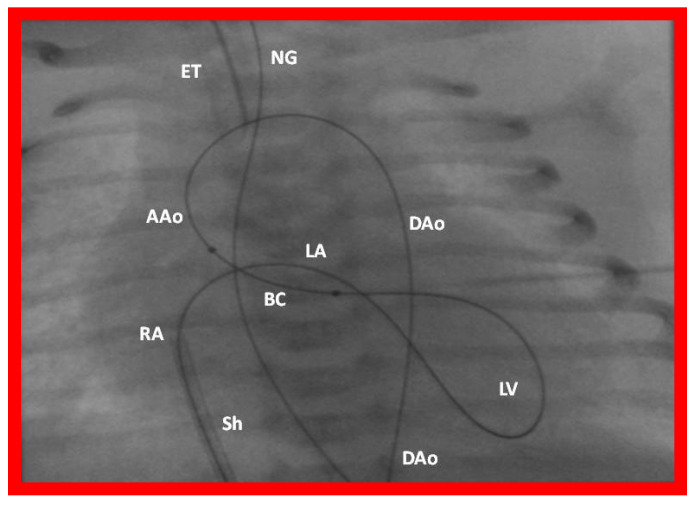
Selected cine-radiographic frame demonstrating the course of the guide wire from the umbilical vein (not shown) to the right atrium (RA), left atrium (LA), left ventricle (LV), ascending aorta (AAo), and descending aorta (DAo). The balloon catheter (BC) is positioned across the aortic valve without the use of a snare as shown in Figure 29 because of easy tack-ability of the Tyshak II catheter used in this case. The sheath (Sh) is seen in the RA, positioned via the umbilical vein. ET, endo-tracheal tube; NG, naso-gastric tube.
